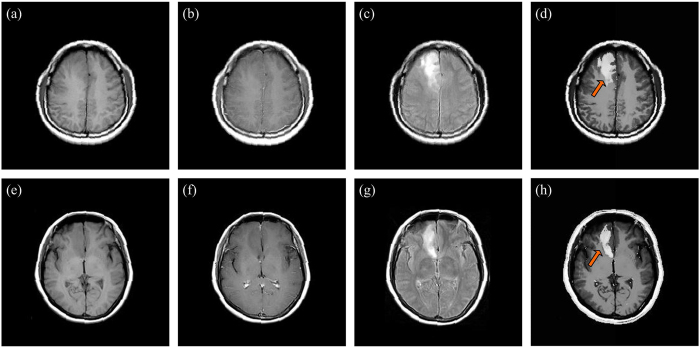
Original and enhanced images obtained by using our method. (a,e), (b,f), (c,g), and (d,h) Original T1-weighted, contrast-enhanced T1-weighted, T2-FLAIR, and enhanced results using the AIFE method.
